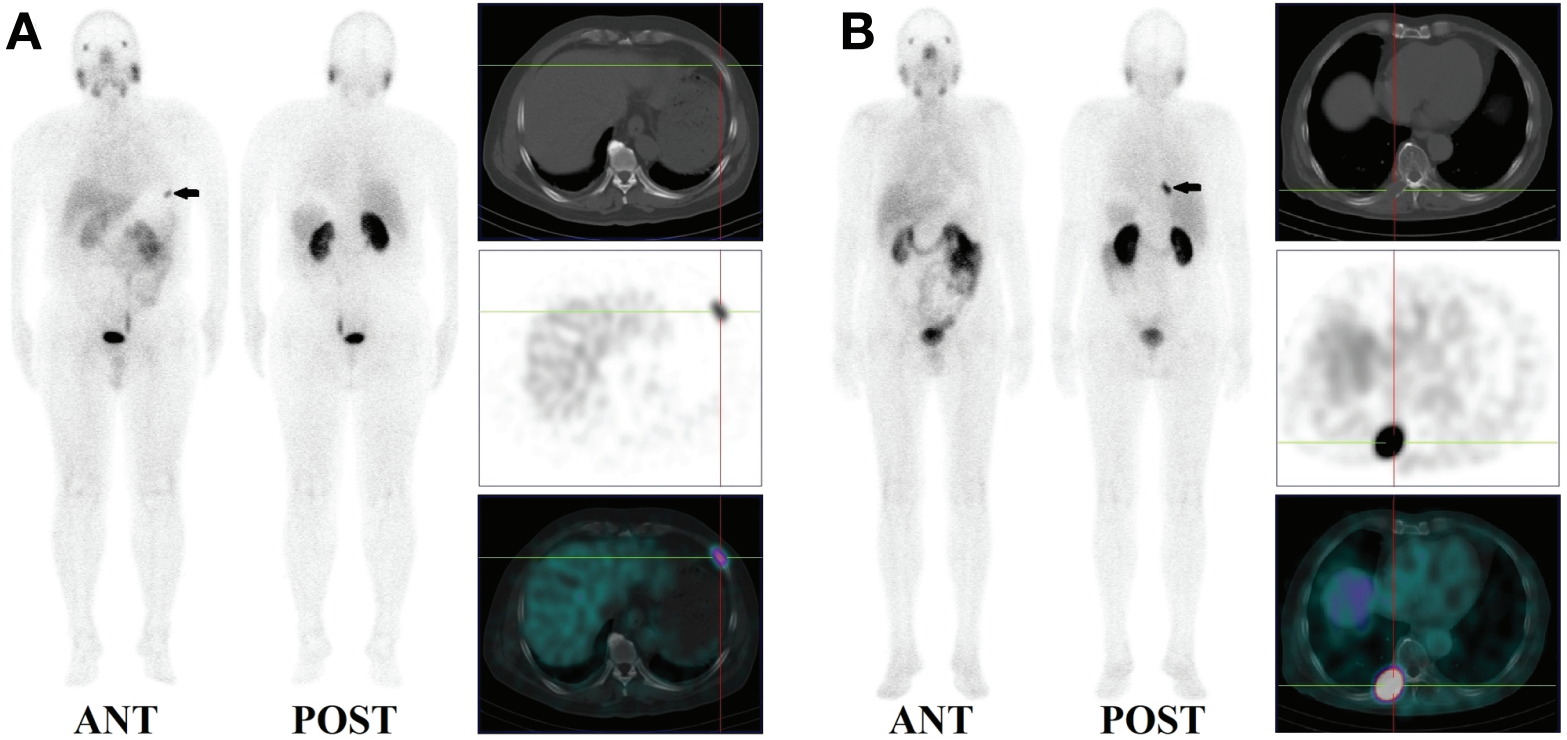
(A): Images of biochemically recurrent prostate cancer in a 71-year-old male with increasing PSA levels after radical prostatectomy (PSA, 0.41 ng/ml; Gleason score,3+4). Whole-body planar scintigraphy (pointed by black arrow) and fused SPECT/CT images show high 99mTC-HYNIC-PSMA uptake (SUVmax, 2.97) in the left 6th rib. However, the corresponding CT showed no obvious bone destruction. The patient received ADT, and his PSA levels decreased to 0.10 ng/ml. (B): Images of biochemically recurrent prostate cancer in a 59-year-old male with increasing PSA levels after radical prostatectomy (PSA, 6.30 ng/ml; Gleason score, 5 + 5). Whole-body planar scintigraphy (pointed by black arrow) and fused SPECT/CT images show high 99mTC-HYNIC-PSMA uptake (SUVmax, 8.63) in the right 8th rib, the corresponding CT showed obvious bone destruction. The patient received radiation therapy and his PSA level decreased to 0.31 ng/ml.
